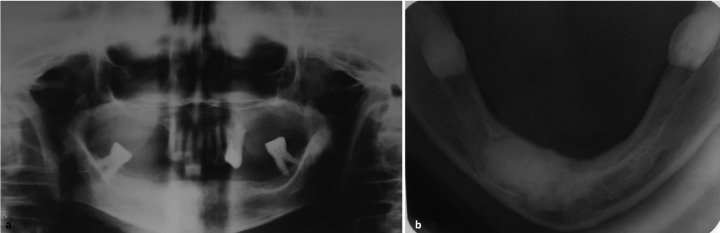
One year postoperative Orthopantomogram a) and b) mandibular occlusal view.
